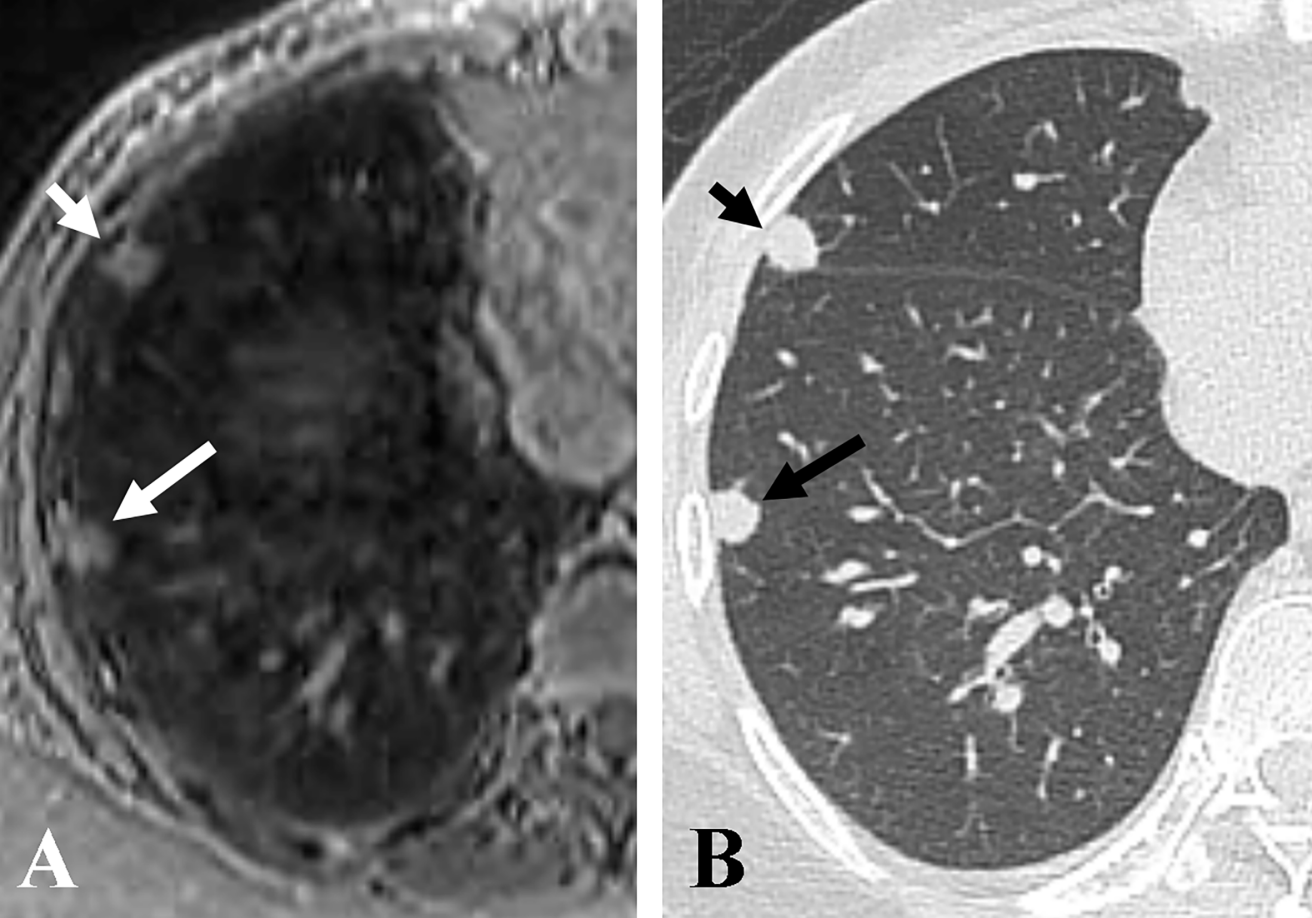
Representative axial images of accelerated spiral 3D UTE (A) and CT (B), showing nodule size underestimation on MRI compared with CT for pleural-based nodules. The overall image quality rating for spiral 3D UTE image was good. The sizes of two pleural-based nodules in the right middle lobe (short arrows) and right lower lobe (long arrows) were 9.5 mm and 7.7 mm on CT, and 8 mm and 6.2 mm on accelerated spiral 3D UTE, respectively.
